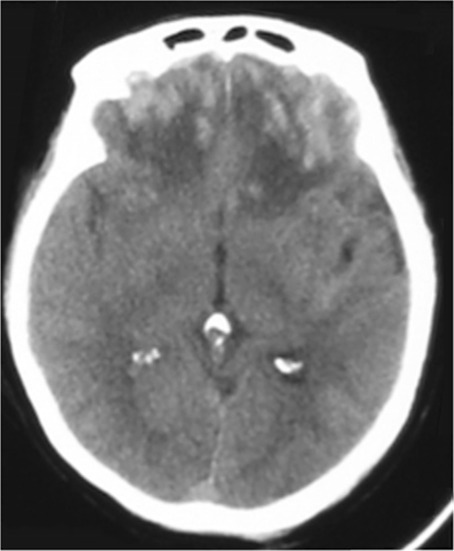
CT image of traumatic bifrontal contusions (adapted from Gao et al. [3])
